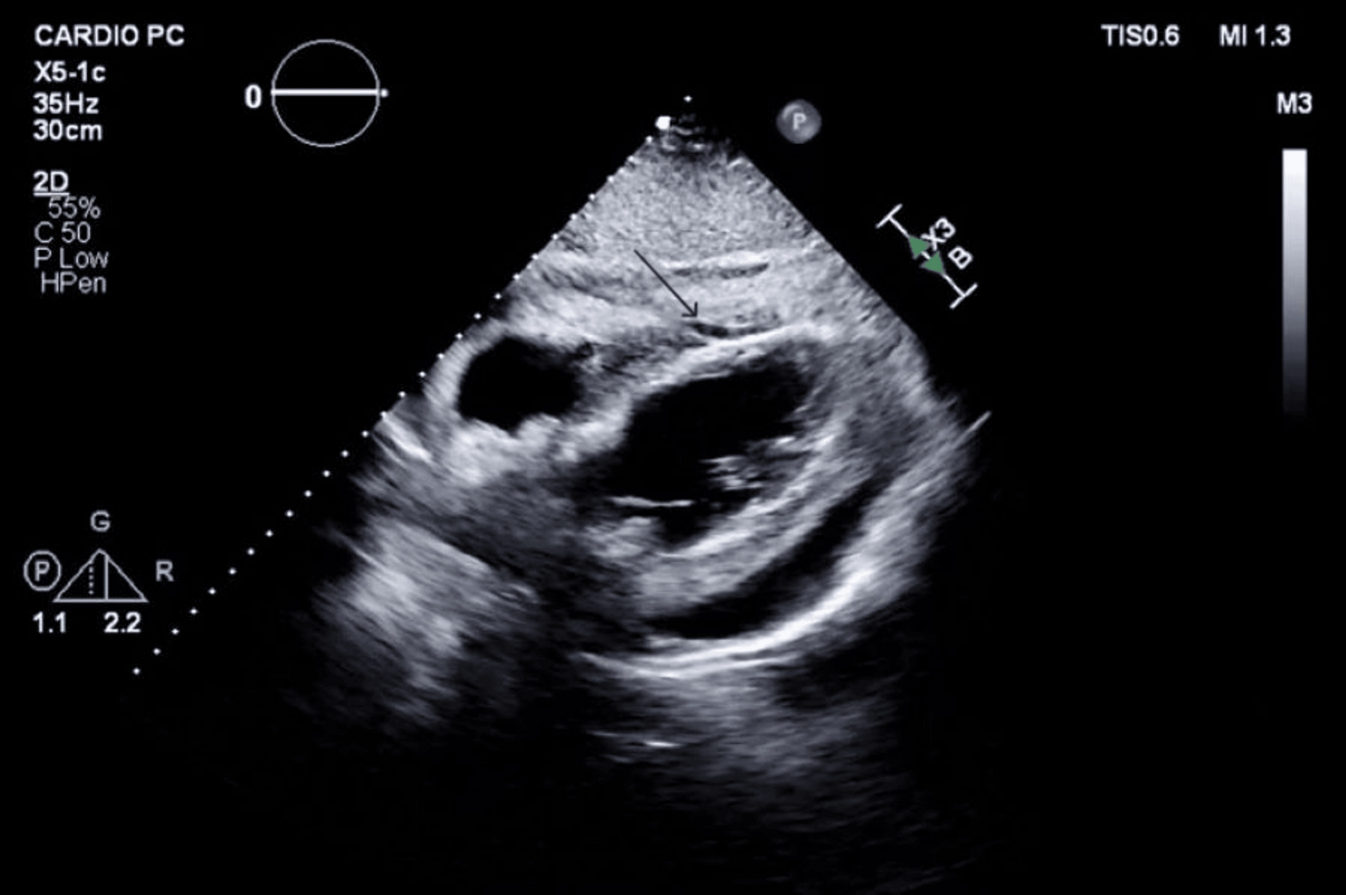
Parasternal window showing moderate pericardial effusion (black arrow)
